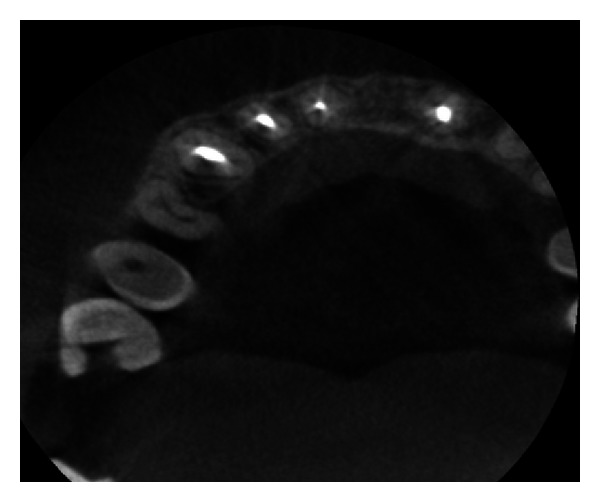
1-year followup CBCT image in axial plane showing establishment of buccal and palatal cortical plates.
